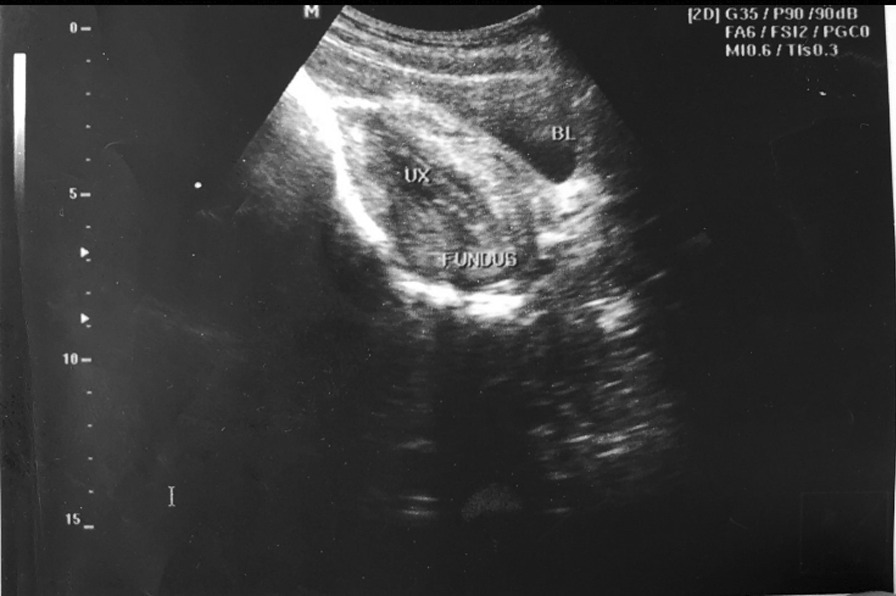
Pelvic ultrasound depicting uterine fundus at cervical OS. UX, Uterus; BL, Bladder; Fundus, Uterine Fundus
